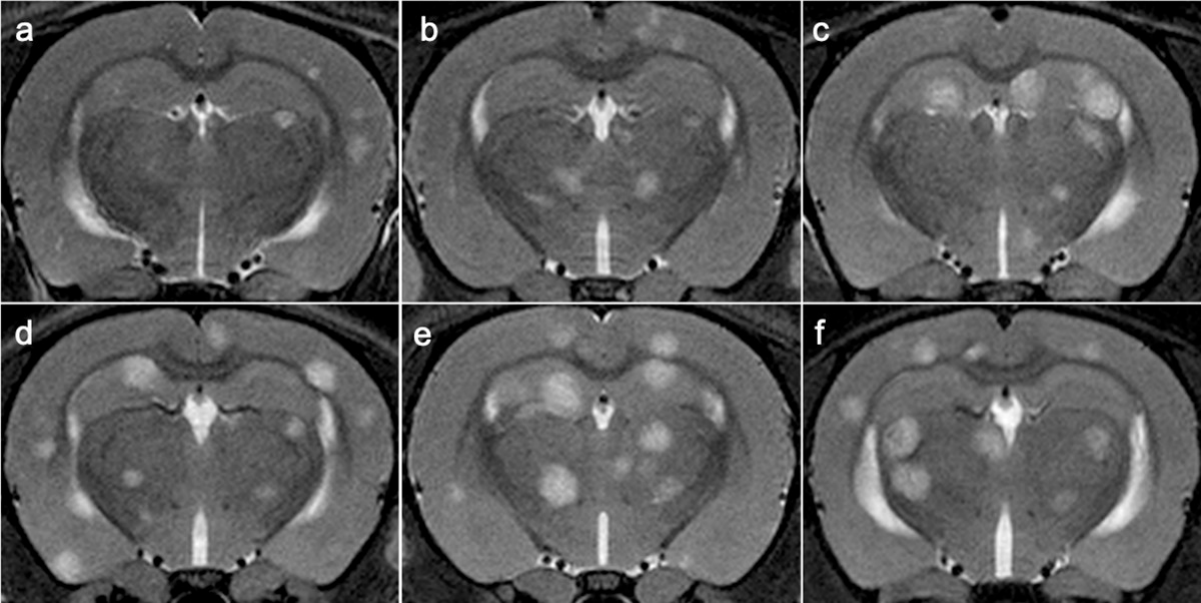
MR imaging of brain metastasis development per passage. a–f. In vivo serial T2w MRI scans showing an increase of brain metastasis development per passage. T2w MR images from a representative case for P1 (a), P2 (b), P3 (c), P4 (d), P5 (e), P6 (f).
